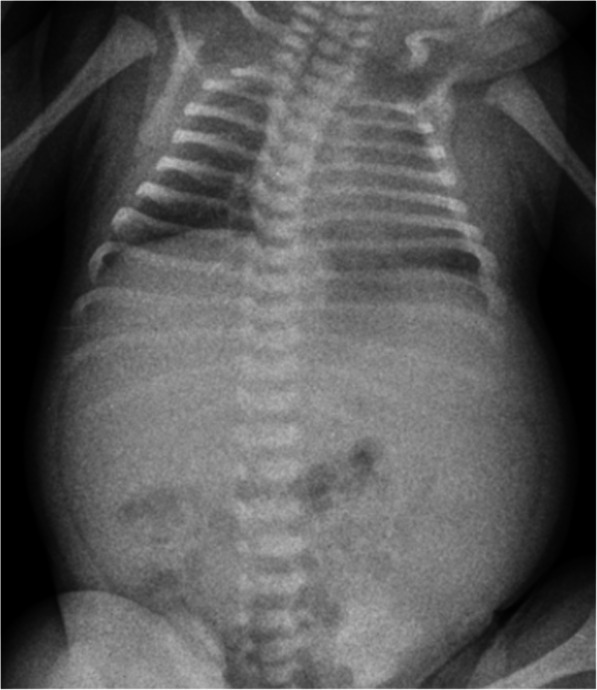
X-ray: lung hypoplasia, with reduced expansion and diffuse bilateral accentuated bronchovascular markings, and severe abdominal distension due to visceromegaly (liver and kidneys)
